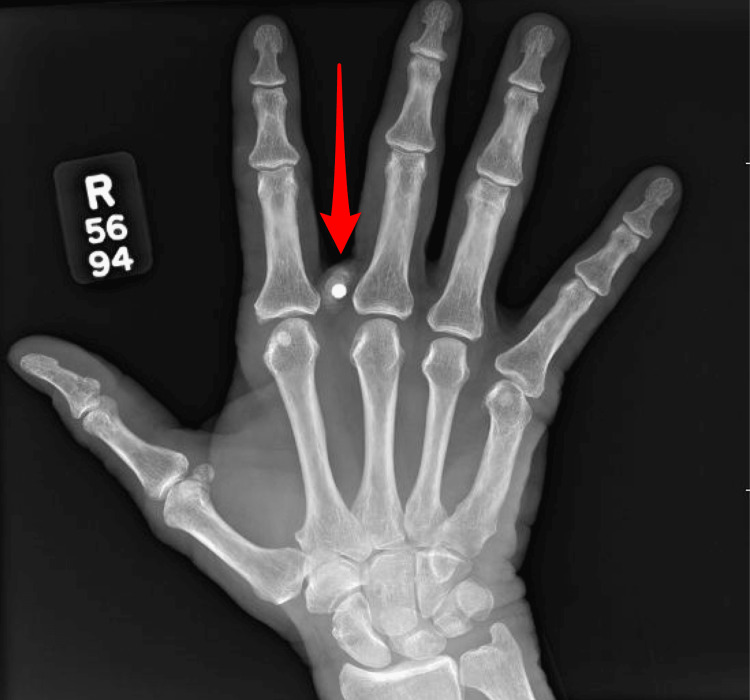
Right hand X-ray with BB pellet before removal.
